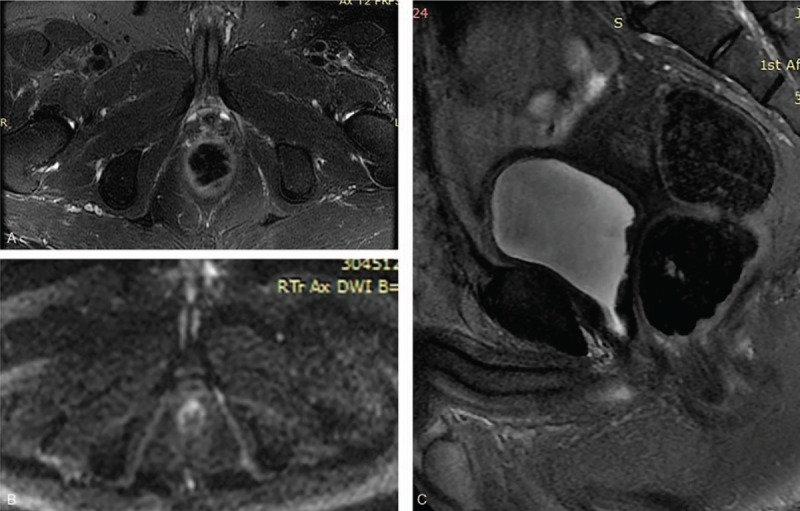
Pelvic MR images (2017-6-26). Follow-up MR images acquired 1 year after SRT, no significant lesion was seen on T2WI MR images (A, C) and DW image (B) on resected prostate tumor bed. SRT = salvage radiation therapy.
